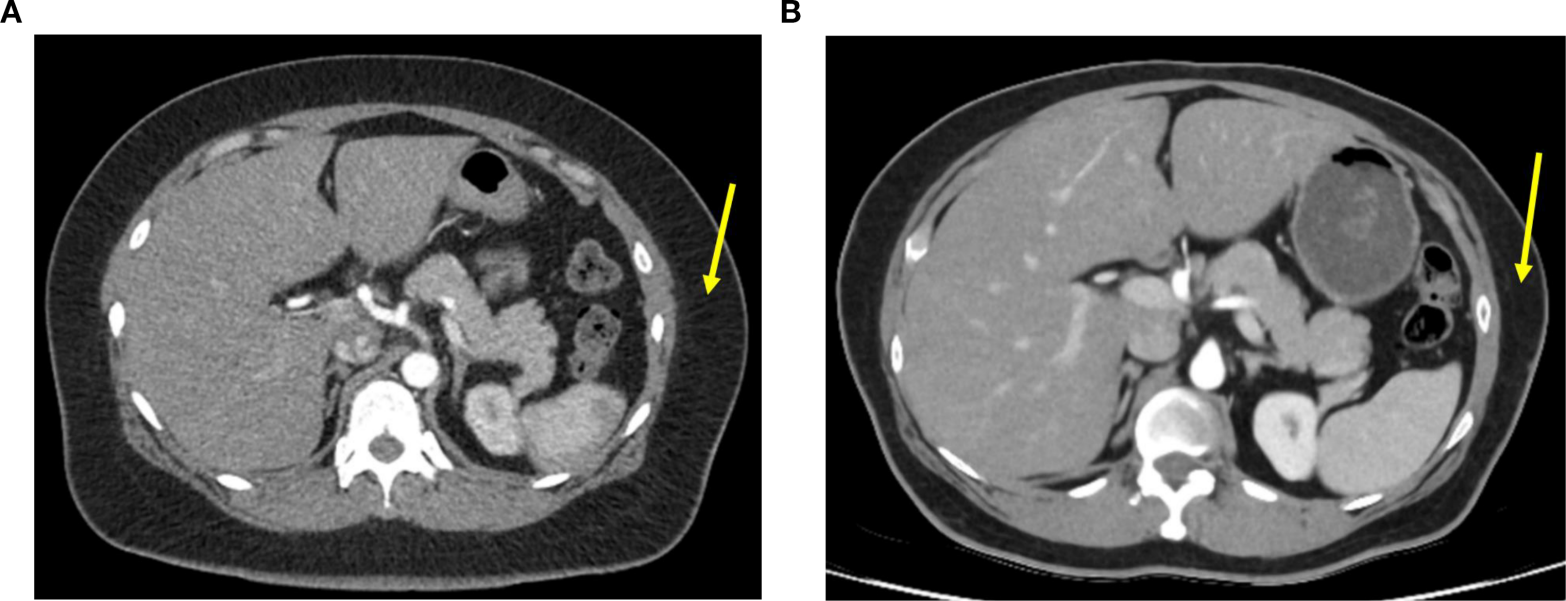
Axial computed tomography slices of the abdomen, demonstrating preserved subcutaneous adipose tissue early after cancer diagnosis in December 2021 (A; *arrow = 35 mm of subcutaneous adipose tissue*); and 30 months later in April 2024 demonstrating loss of subcutaneous adipose tissue (B; *arrow = 20 mm of subcutaneous adipose tissue*).
